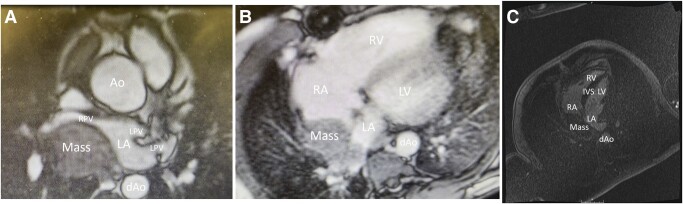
(*A*) Steady-state free precession cardiac magnetic resonance imaging showing the mass compressing the posterior left atrium and right pulmonary vein. (*B*) First-pass perfusion cardiac magnetic resonance imaging showing enhancement of the mass, suggesting hypervascularity. (*C*) Delayed enhancement cardiac magnetic resonance imaging signifying the presence of a rich stroma and neovascularized mass. Ao, aorta; dAo, descending aorta; IVS, interventricular septum; LA, left atrium; LPV, left pulmonary vein; LV, left ventricle; RA, right atrium; RPV, right pulmonary vein; RV, right ventricle.
